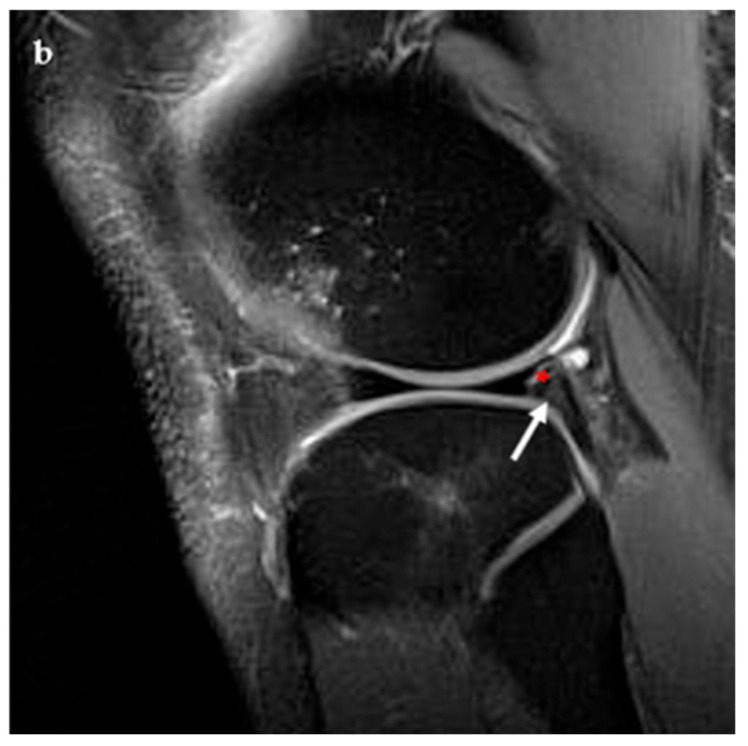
(a) Axial proton density-weighted 1.5-T MRI image and (b) sagittal proton density-weighted 3-T MRI image demonstrating the popliteus hiatus (red asterisk) and the popliteus tendon (arrow) coursing through it.
